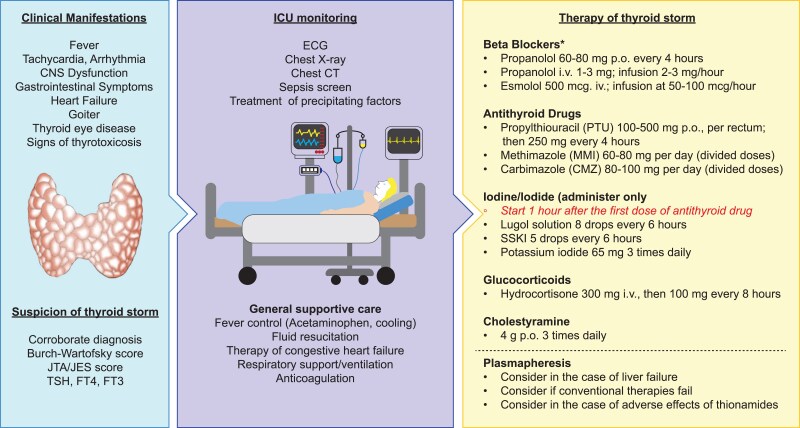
Diagnosis and management of thyroid storm. For beta-blockers, thyroid medications, and iodine, one of the listed options is chosen. *In addition to these commonly used beta blockers, alternative regimens with atenolol, metoprolol, and nadolol per os, as well as landiolol and labetalol intravenously, have been used successfully. For further details, see text and references (5, 6). Abbreviations: CT, computer tomogram; JTA/JES, Japan Thyroid Association/Japan Endocrine Society; p.o., per os; i.v., intravenous; SSKI, saturated solution of potassium iodide.
